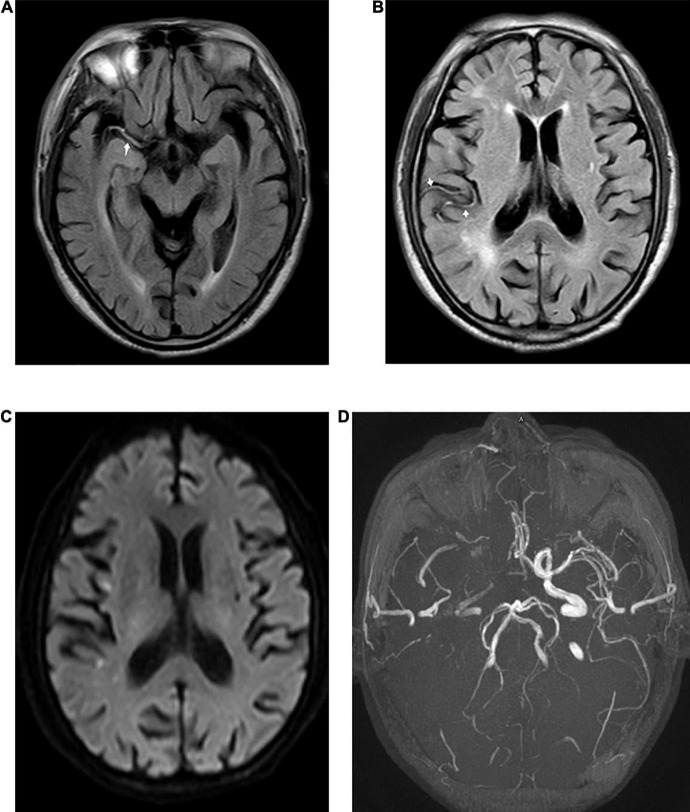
Fluid-attenuated inversion recovery (FLAIR) vascular hyperintensity (FVH) detected in a 73-year-old male patient with acute stroke. Proximal FVH in the Sylvian fissure [(A), arrow] and distal FVH [(B), arrow] in the right temporal lobe were detected in FLAIR images. Diffusion-weighted imaging (DWI) (C) shows acute ischemic infarction in the territory of the right middle cerebral artery (MCA). A time-of-flight magnetic resonance imaging (MRI) sequence (D) shows the right MCA and an occlusion of the internal carotid artery (ICA).
